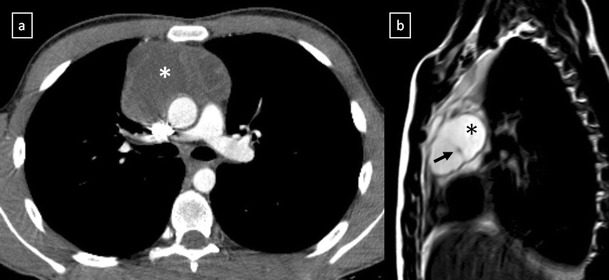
Acquired thymic cyst in a 43-year-old man. a Contrast-enhanced CT scan shows a well-defined water-attenuation multiloculated mass (*) in the anterior mediastinum. b Sagittal T2-weighted MR sequence demonstrates a multiloculated mass with typical high signal intensitiy and fine internal septa within (*arrow*)
